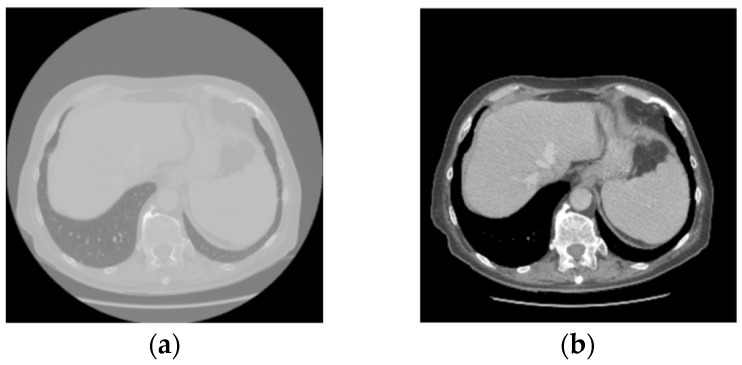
CT image processing performed to enhance the visibility of the liver and tumor regions by normalizing the Hounsfield Unit values to the range of −250 to 250: (a) the original CT slice; (b) the enhanced CT slice.
